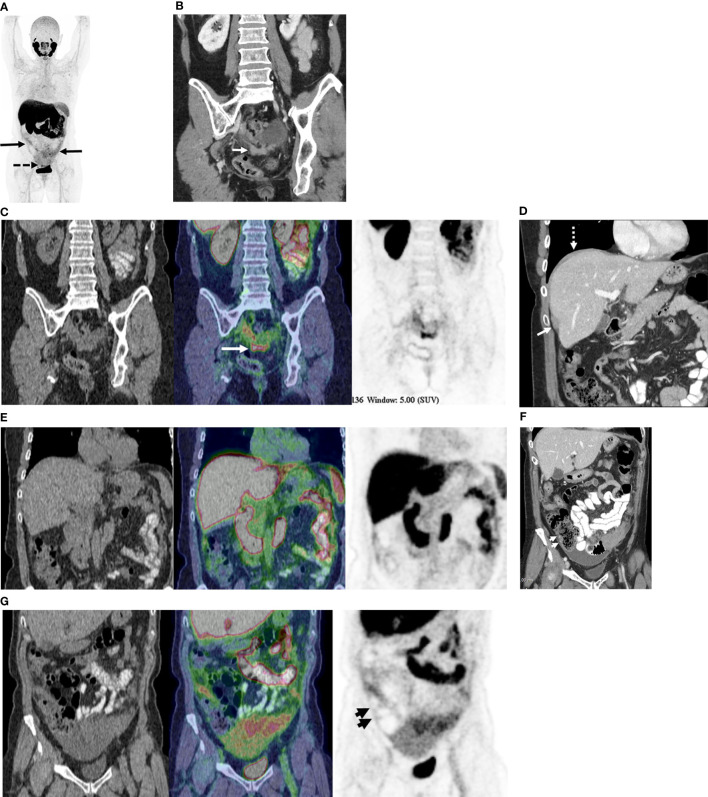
68-year old with stage IIIC high-grade serous ovarian cancer. (A) Maximum Intensity Projection image of 18F-DCFPyL shows mild to moderate radiotracer uptake in omental metastases (solid arrows) and moderate radiotracer uptake in pelvic peritoneal metastases (dotted arrows). (B) Coronal contrast enhanced CT image shows peritoneal deposits in the posterior cul-de-sac (arrow). (C) Coronal PET/CT image (CT - left, fused PET/CT image – middle; PET – right) corresponding to B shows moderately radiotracer uptake in same metastatic deposit (concordant CT and PET). Metastatic disease was confirmed at surgery. (D) Coronal contrast enhanced CT image shows metastatic disease on right diaphragm (dotted arrow) and along capsular surface of liver (solid arrow). (E) Coronal PET/CT image (CT - left, fused PET/CT image – middle; PET – right) corresponding to D show no focal radiotracer uptake visible on right diaphragm or liver capsule. Surgical pathology confirmed CT findings of metastatic disease at these sites. (F) Coronal contrast enhanced CT image shows focal thickening along right lateral wall of ascending colon (short arrows), suspected to represented serosal metastasis. (G) Coronal PET/CT image (CT - left, fused PET/CT image – middle; PET – right) corresponding to F shows no focal radiotracer uptake on the serosal surface of the ascending colon. No serosal disease on surface of the right colon was found at surgery.
